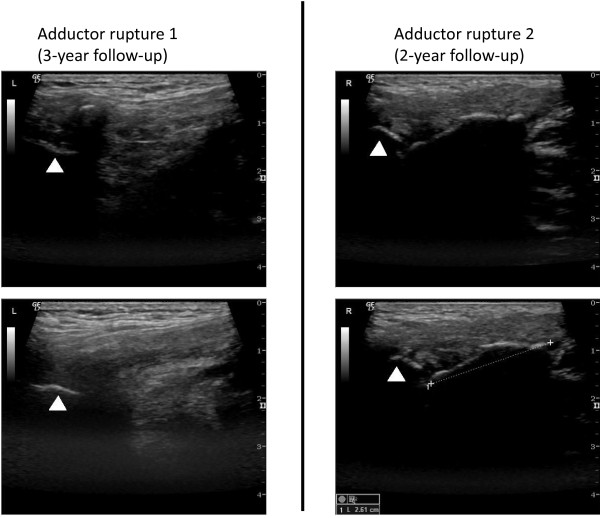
Ultrasonographic assessment at 2-year and 3-year follow up following adductor longus ruptures. Adductor rupture 1 (3 years): White arrow (Insertion), Small calcification in the tendon. Adductor rupture 2 (2 years): White arrow (Insertion), Calcifications (2.6 cm).
